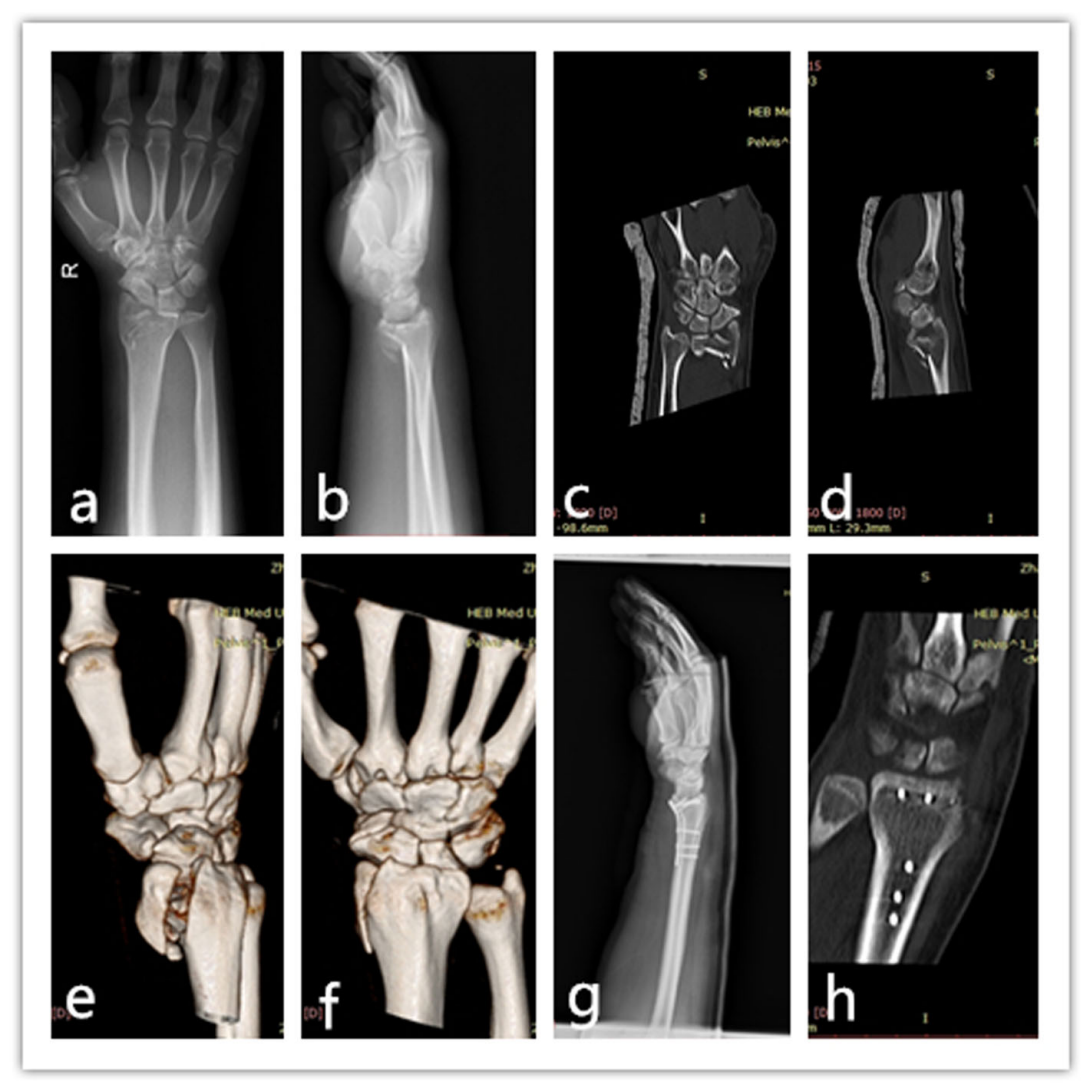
Patient, male, 39 years old, suffered from motor collision accident and sustained fracture in his left wrist. The preoperative radiographs (a, b) and CT scanning (c–e) showed the fracture of lunate facet with slight subsidence); and the fracture line extended to metaphysis. He underwent external fixation treatment (f–h) and the CT scanning showed the favorable wrist joint congruence
